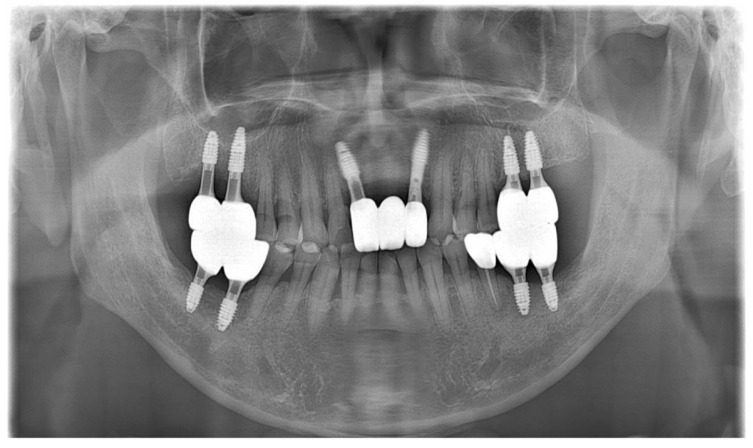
Panoramic radiograph after 2 years.
